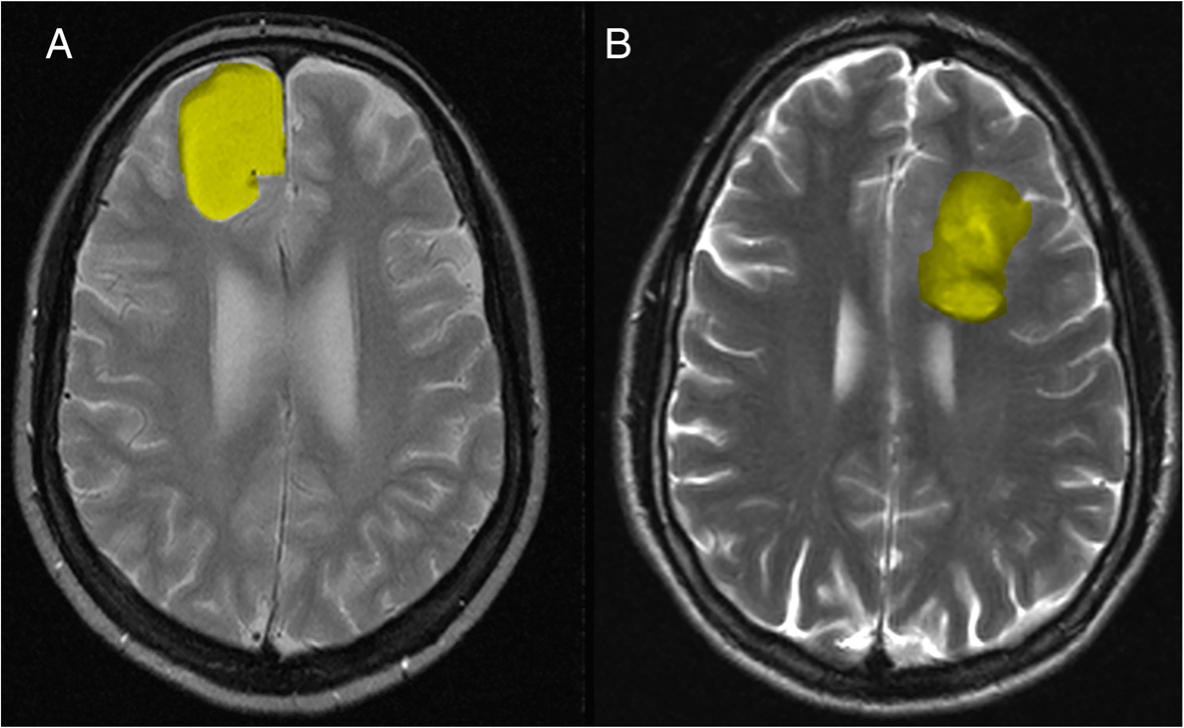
Tumor (T2-weighted MR images) localization in the cortex without subventricular zone involvement (a). Tumor (T2-weighted MR images) with infiltration of the subventricular zone (b)
